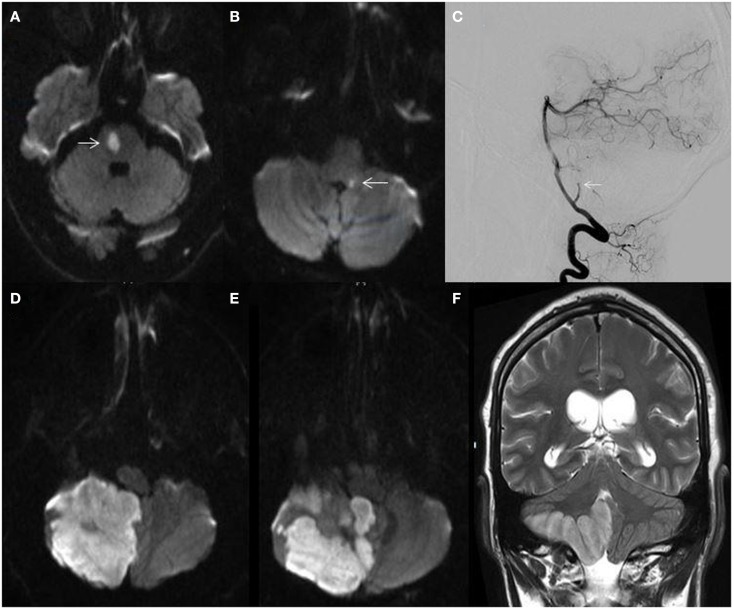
(A) MRI diffusion-weighted image (DWI) demonstrating a right ventral pontine infarction (arrow) in a 62-year-old man with fluctuating left sided weakness; (B) MRI-DWI showing a small dorsal left medullary infarction (arrow) in a 58-year-old man with hypertension and hyperlipidemia presenting with acute isolated vertigo; (C) catheter angiogram showing cut-off of the right posterior inferior cerebellar artery (arrow); (D,E) MRI-DWI showing massive right cerebellar hemispheric and vermian infarction; (F) MRI T2-weighted sequence demonstrating right cerebellar infarction with edema and mass effect.
